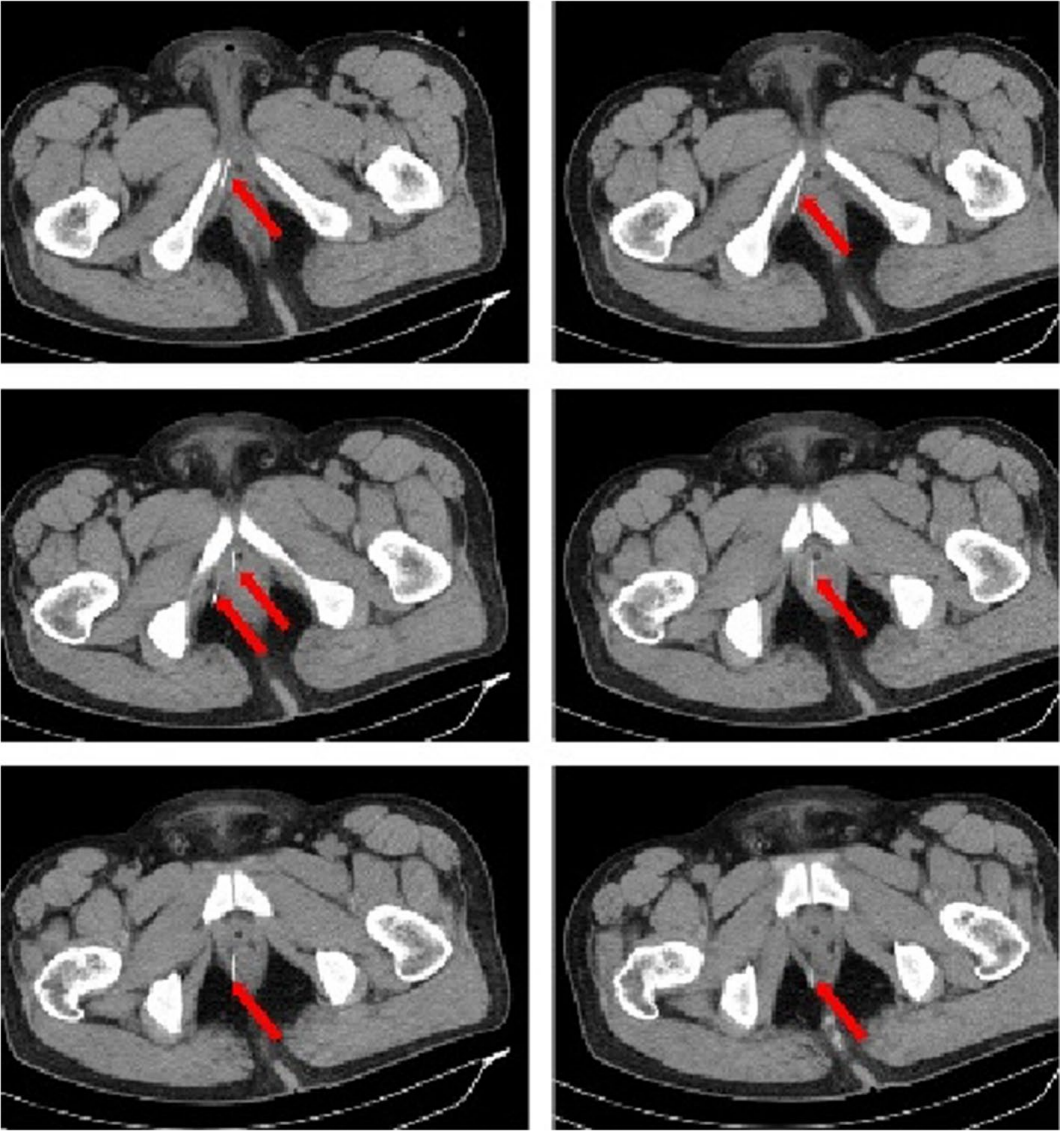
July 27, 2023, CT of the pelvis. The arrows point to the needles
